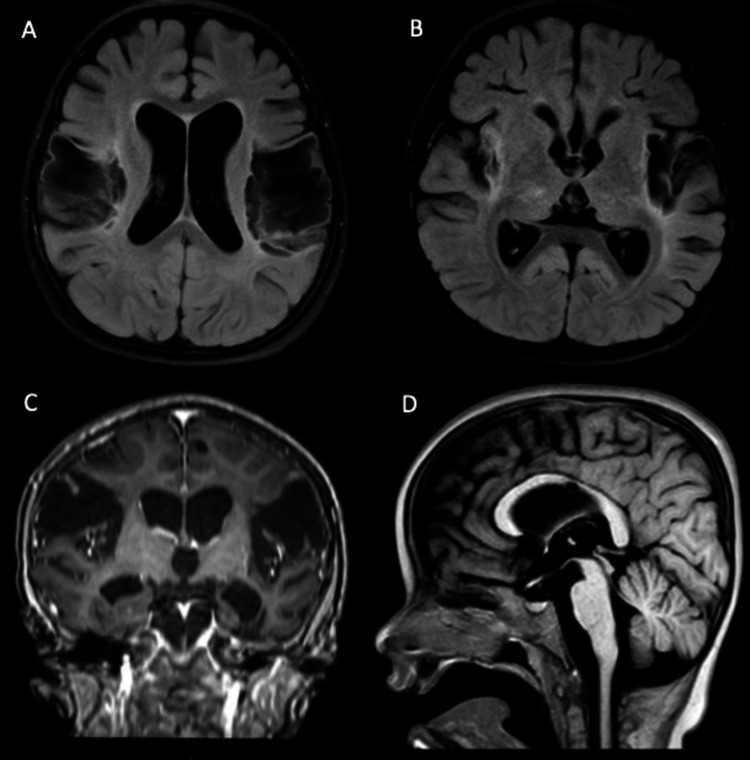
Follow-up brain MRI after one year of presentation. A: Axial T2 FLAIR. B: Axial T2 FLAIR. C: Coronal MPR. D: Sagittal T1 FLAIR. FLAIR, fluid-attenuated inversion recovery; MPR, multiplanar reformation.
